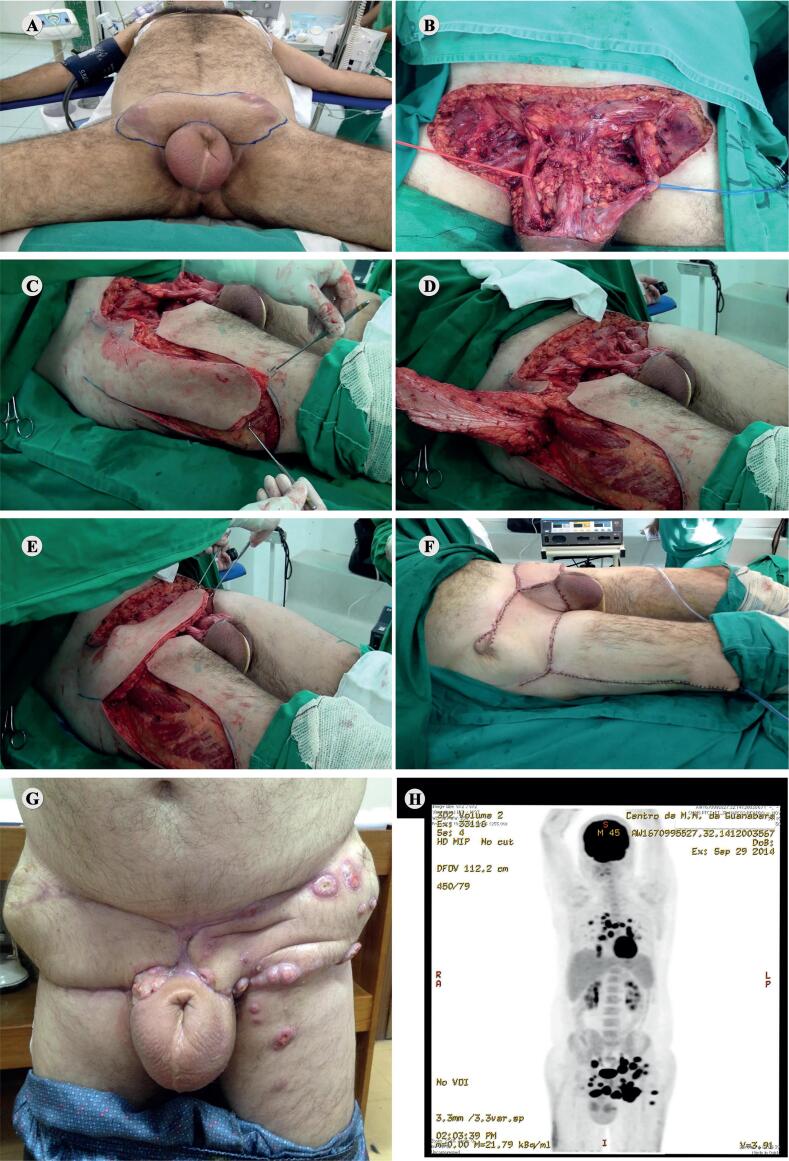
A) Patient with bulky bilateral inguinal lymphadenopathy who lost follow-up after partial penectomy, in supine position, with legs fixed in moderate external rotation, prepared for surgical approach. B) Large wound defect produced after bilateral primary radical inguinal surgical debulking (MPFR). C, D and E) Step by step bilateral MPFR using tensor fascia lata (TFL) flap. F) Final aspect of the tissue reconstruction using bilateral TFL flap. G) Local disease recurrence with cutaneous involvement of the TFL flap. H- Pet CT evidencing multiple secondary implants in the inguinal, pelvic and thoracic regions.
